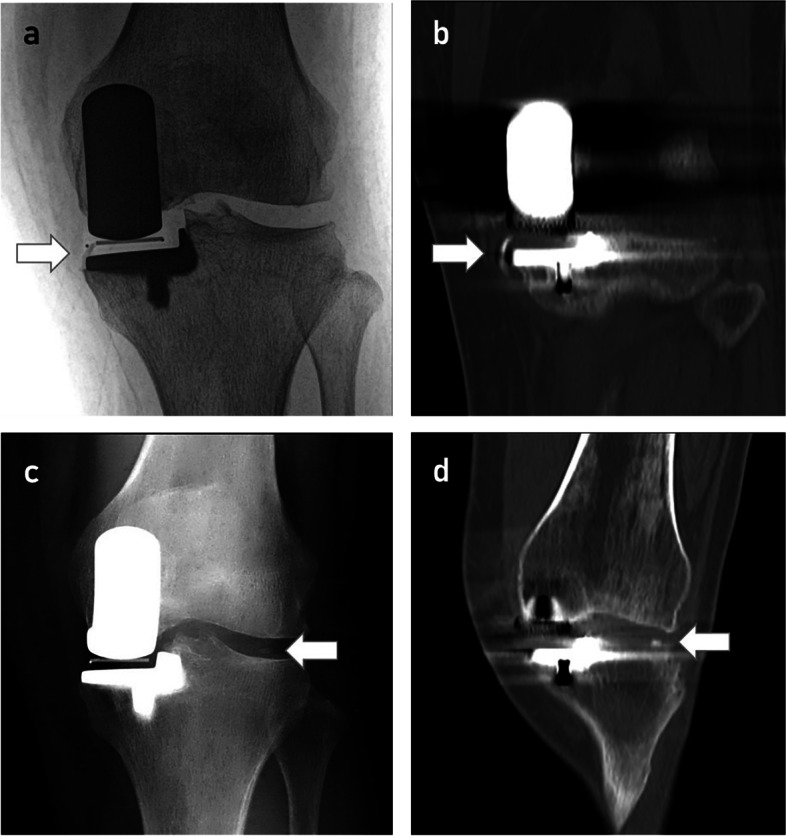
A.B. Postoperative standard unicompartmental fluoroscopy and CT showed residual cement (arrow) in the medial tibial prosthesis. C. D. When the patient developed symptoms 2 years after surgery, radiology revealed that the foreign body in the lateral compartment (arrow) was the same as the previous medial cement residue
